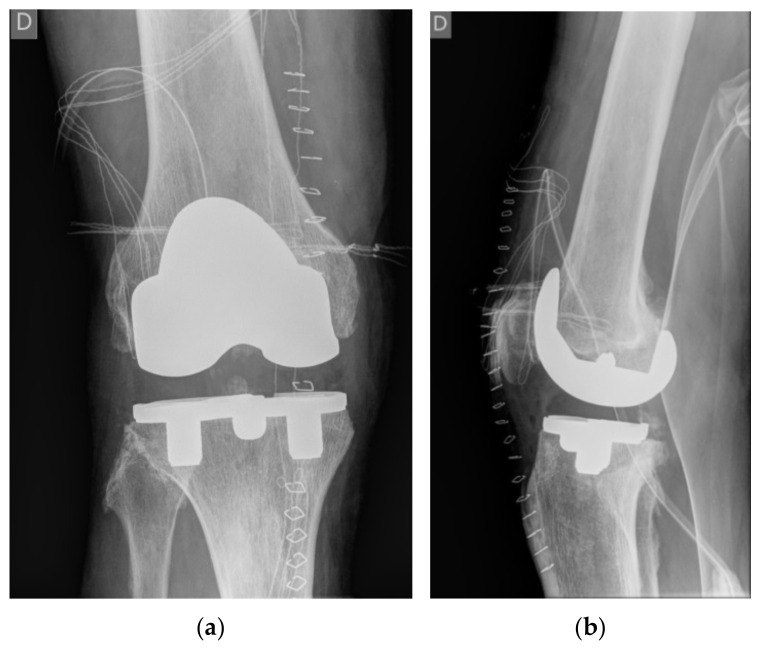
Anteroposterior (a) and lateral (b) radiographs of the right knee on the day of surgery after inserting the total uncemented endoprosthesis with modular porous tantalum tibial tray.
